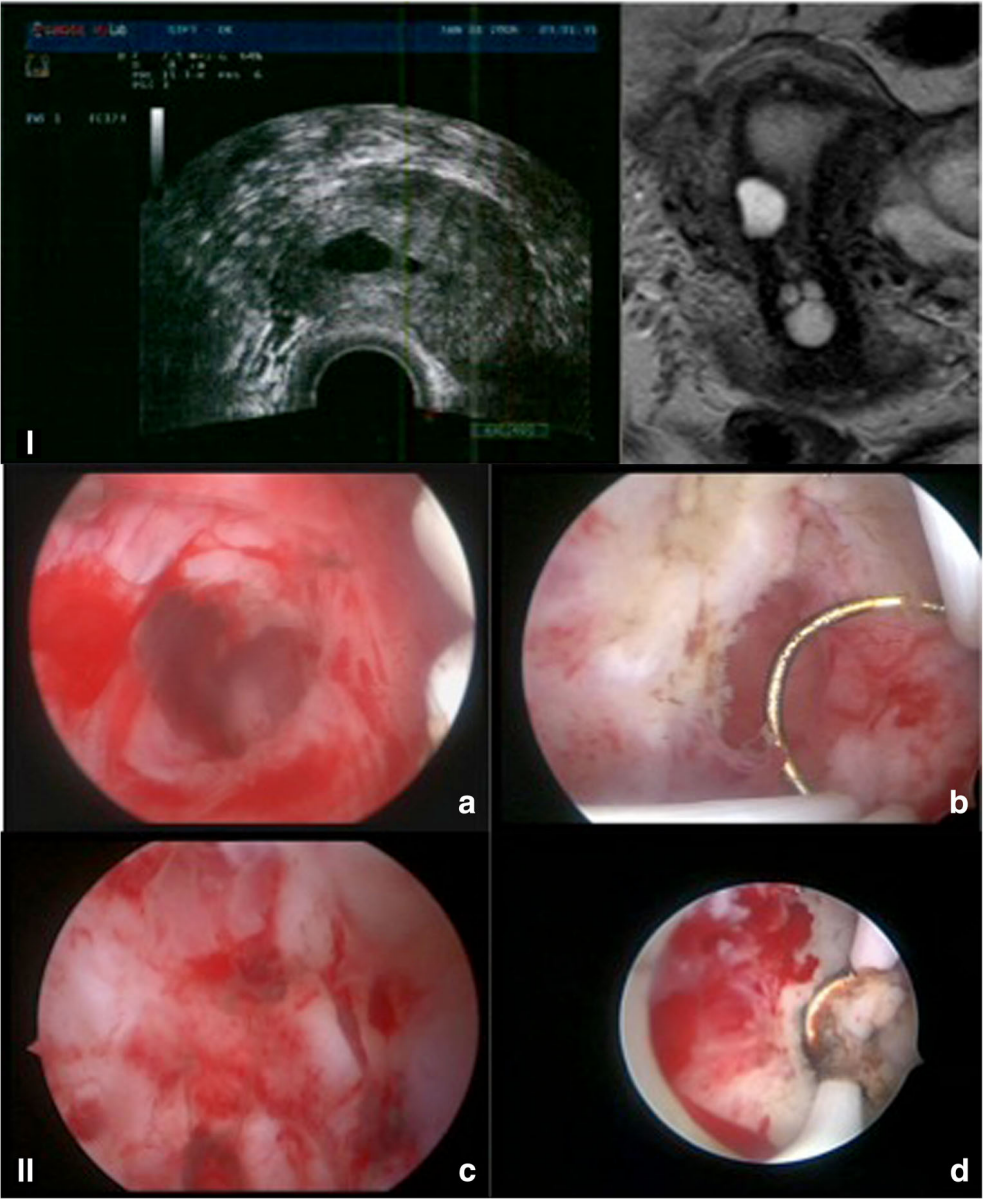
Case no. 2. I Ultrasound and MRI image of cystic lesion right isthmic part. II
